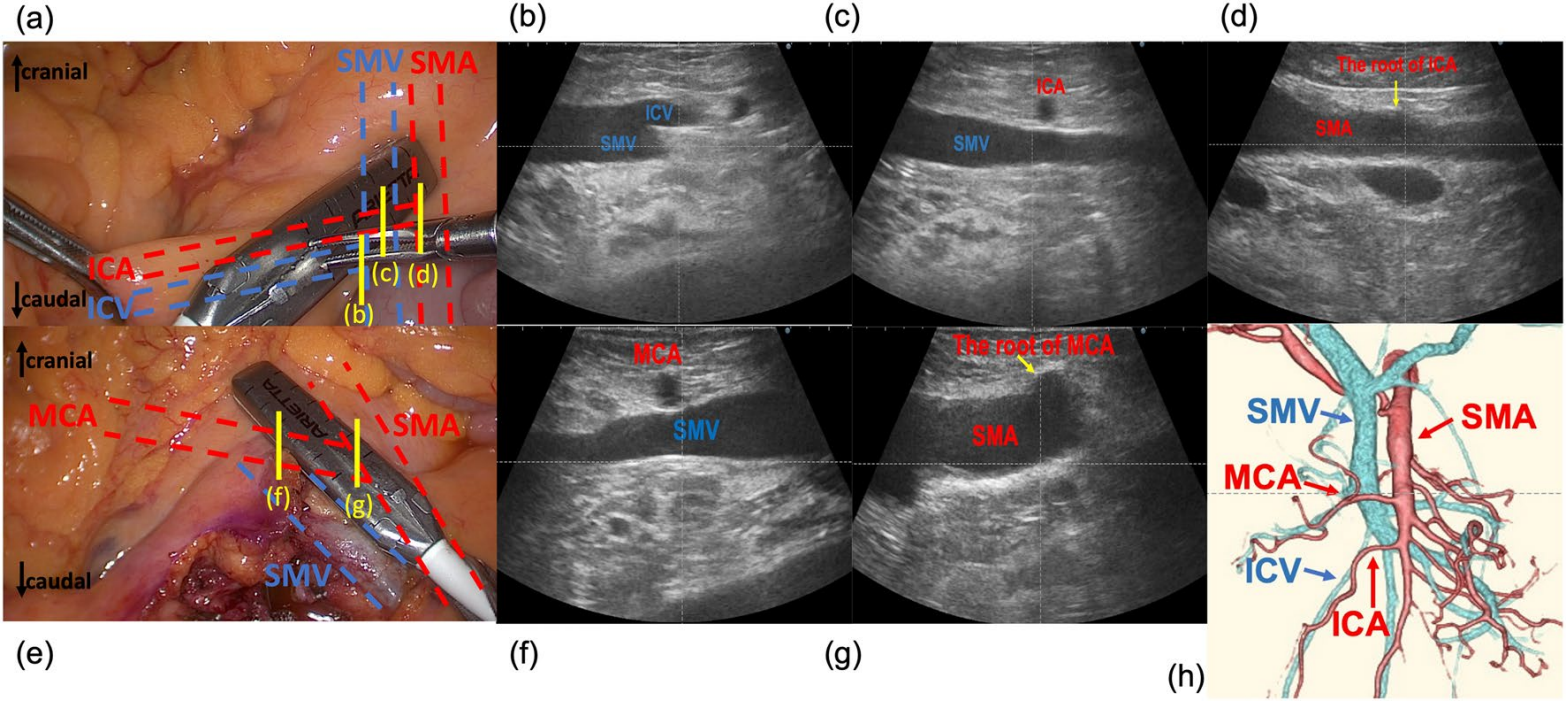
Procedures of intraoperative ultrasound examination for RSCC. (a–d) 1st scan: (a) the intraoperative image of 1st scan. The SMA and SMV, which pulled lateral side by the assistance, were scanned from their periphery toward the roots. (b) at the root of the SMV where ICV was branched. (c) above the SMV crossed by the ICA. (d) at the root of the SMA where ICA was branched. (e–g) 2nd scan: (e) the intraoperative image of 2nd scan. The transverse mesocolon was pulled cranial side by the assistance, and MCA was scanned from its periphery toward the root. (f) Above the SMV crossed by the MCA. (g) At the root of the SMA where MCA was branched. (h) 3D-CT angiography showed the ICA and MCA anterior to the SMV. SMA superior mesenteric artery, SMV superior mesenteric vein, ICV ileocolic vein, ICA ileocolic artery, MCA the middle colic artery, 3D-CT three- dimensional computed tomography.
